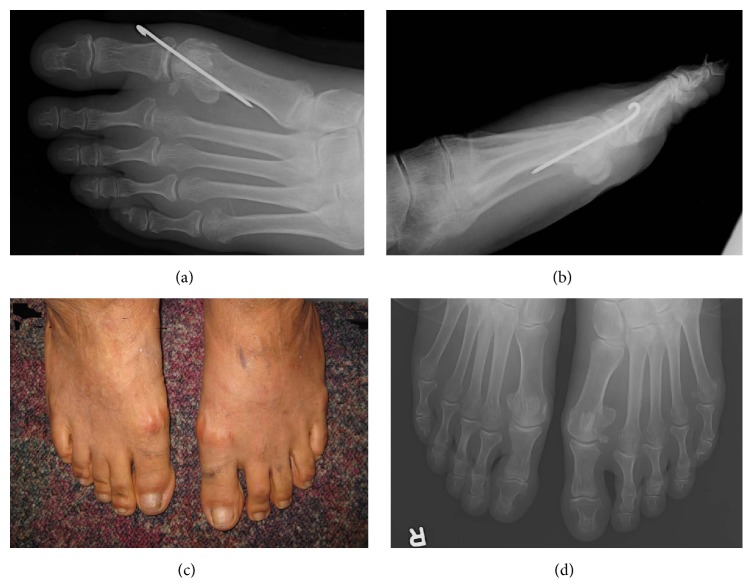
(a, b) Postoperative X-rays showed the correction of the hallux varus and the K wire in situ. (c) Clinical photos 31 months after the operation showed the correction maintained. (d) Radiograph showed the correction maintained and no degenerative change of the 1st MTPJ 31 months after the operation.
